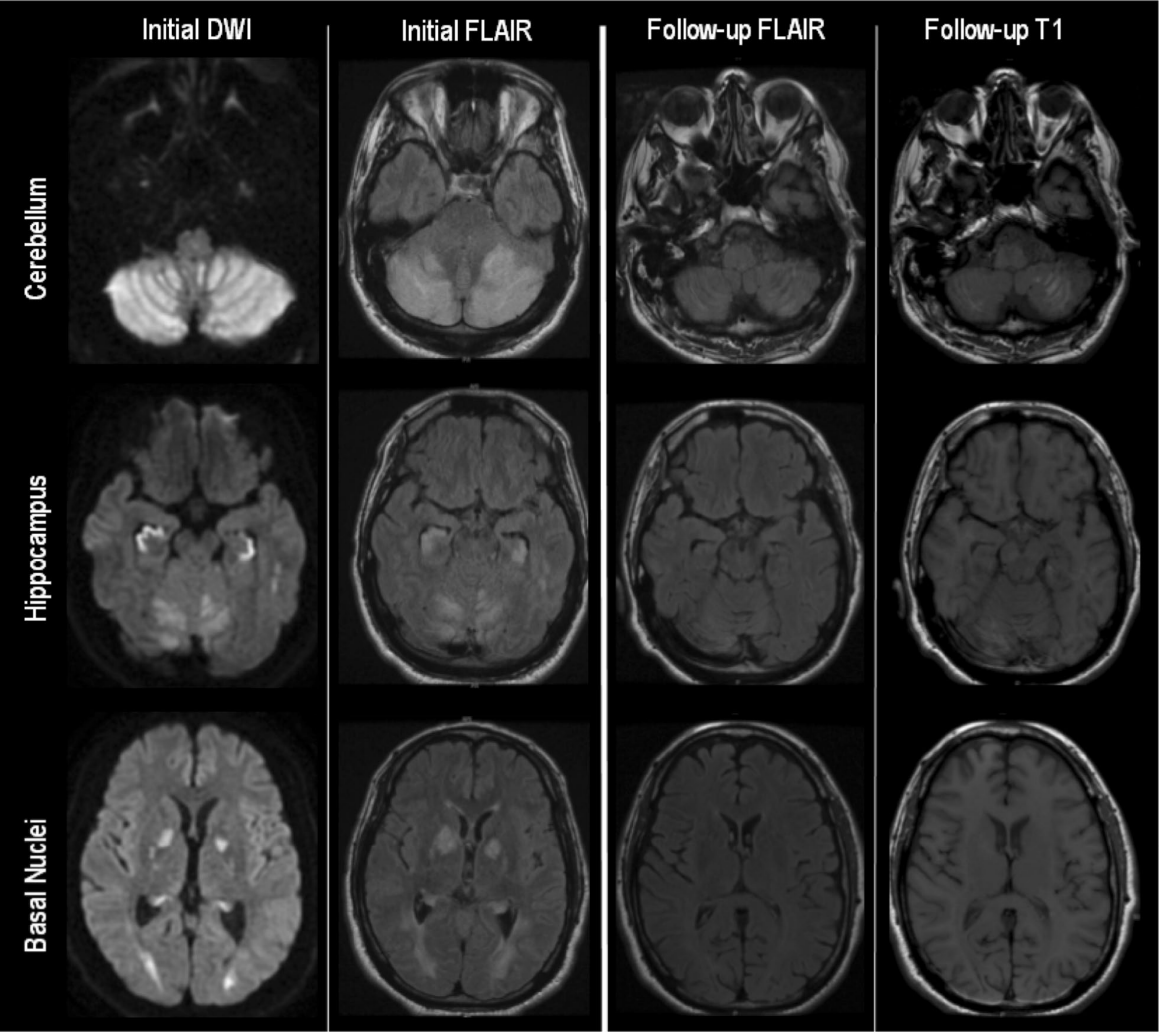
Patient #5 MRI brain, selected sequences, initial and three-month follow-up. *DWI* diffusion-weighted imaging, *FLAIR* fluid-attenuation inversion recovery, *MRI* magnetic resonance imaging. Evolution of imaging changes between initial presentation and repeat imaging 3 months later. Abnormal restricted diffusion was not present on follow-up imaging (not shown)
